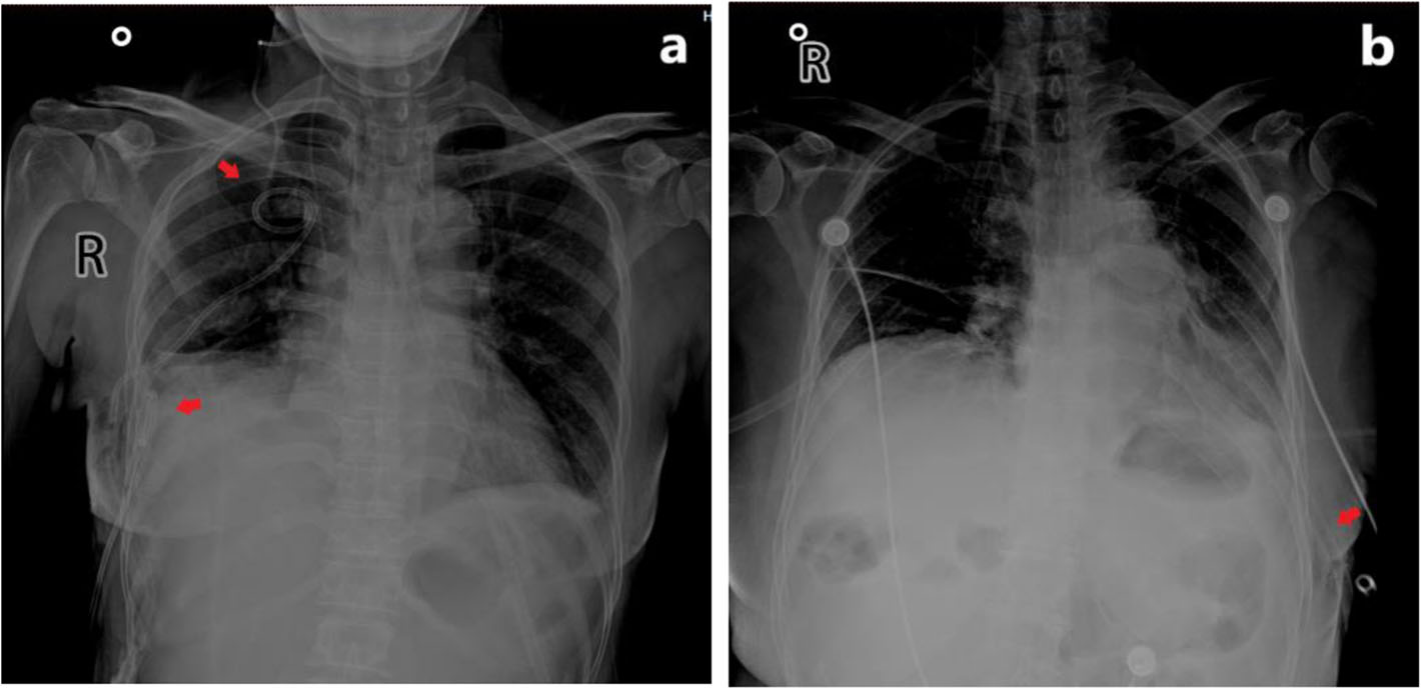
Postoperative X-ray of the thoracic cavity of patients; a the arrows indicate that two 8F chest drainages tubes are placed in the thoracic cavity; b the arrows indicate that one 24F chest drainage tube is placed in the thoracic cavity
